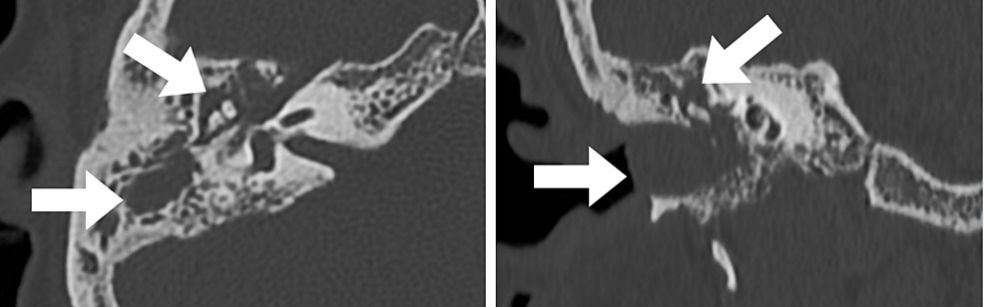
Axial and coronal reconstructed HRCT images of the right temporal bone show soft tissue thickening in the right middle ear cavity and mastoid air cells associated with bony erosions. HRCT: high-resolution computed tomography.
